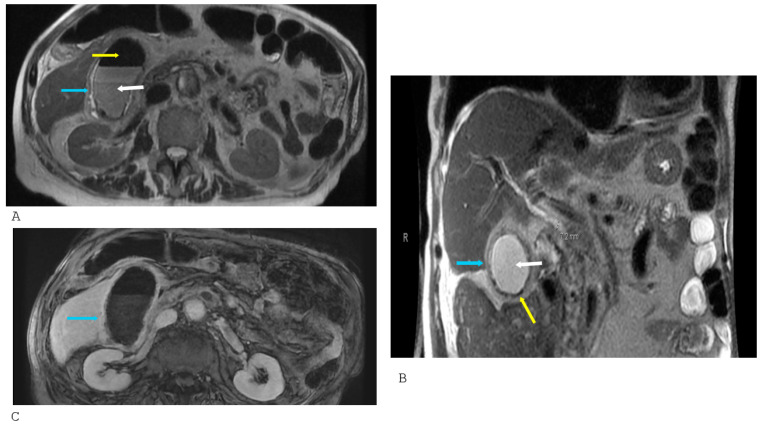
Magnetic resonance imaging of a 53-year-old female diabetic patient with emphysematous cholecystitis. (A,B): Axial T2 and coronal T2 sequences demonstrate gallbladder sludge (white arrow), intraluminal gas and intramural gas signified by the gas-related signal voids (yellow arrows), and gallbladder wall edema (blue arrow); (C): Post-contrast axial sequence demonstrates mucosal hyperenhancement (blue arrow) of the gallbladder wall. The lack of enhancement of the gas-related signal voids (yellow arrows) and signal void on all sequences distinguishes them from susceptibility and flow-related artifacts, which would enhance.
